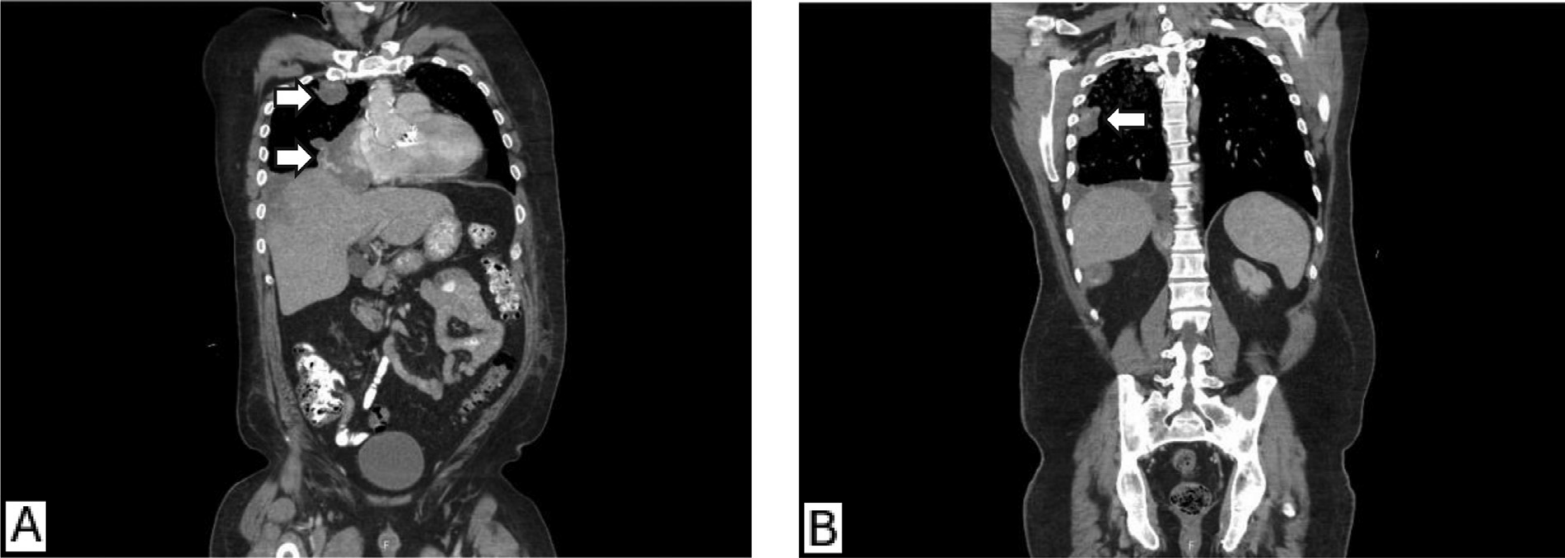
Coronal contrast-enhanced CT chest: (A) Large heterogeneously enhancing mass (7.6 × 6.8 cm) originating from the right atrial wall and extending along the right heart border. A 3.1 cm pleural metastasis is noted anterior to the right upper lobe. (B) Enhancing anterior right basilar pleural lesion (4.5 cm) with interval reduction in right pleural effusion and improvement in pulmonary ground-glass opacities.
